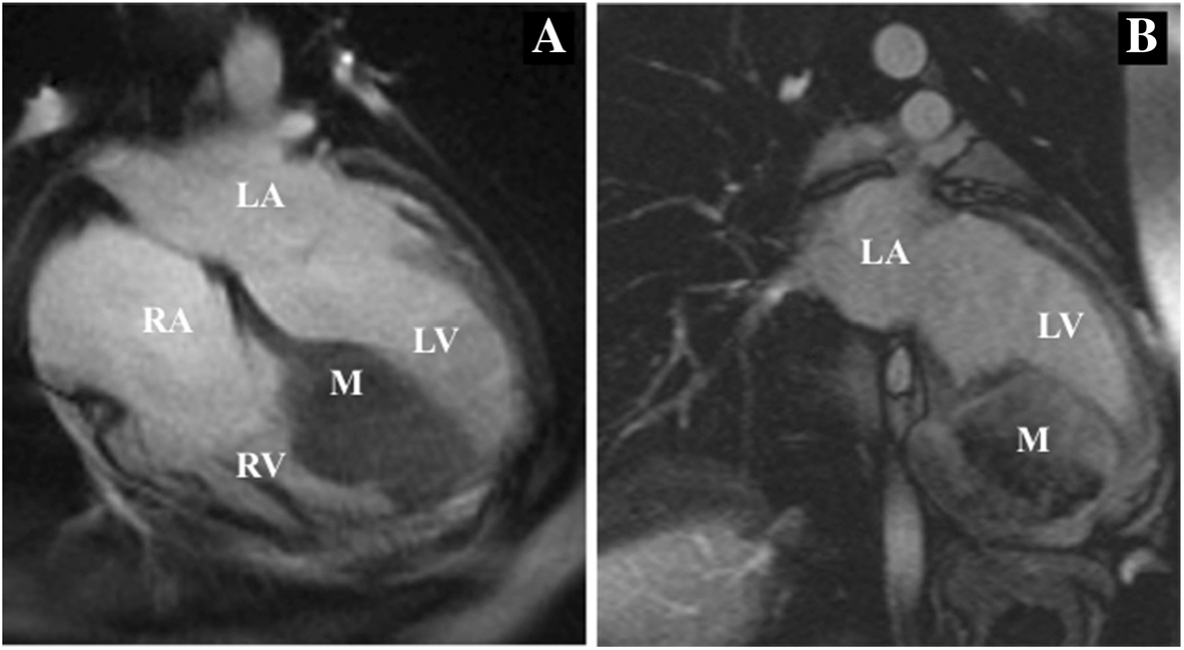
Cardiac magnetic resonance images. a A low-density mass located in the intraventricular septum on the T2-weighted image. b The mass involved the apex and the posterior wall of the left ventricle (LV) and oppressed the LV cavity. *RA* right atrium, *RV* right ventricle, *LA* left atrium, *LV* left ventricle, *M* mass
